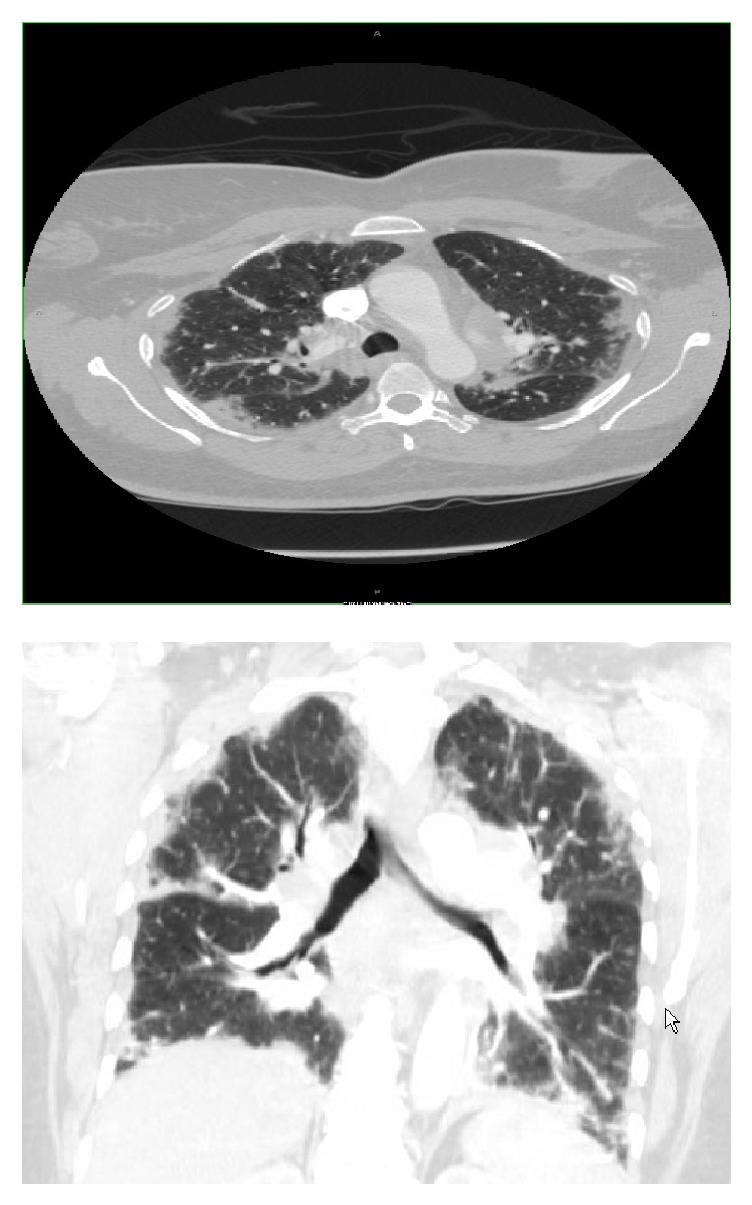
Chest computed tomography (CT) scan showing increased bilateral interstitial lung markings with bilateral hilar and mediastinal lymphadenopathy most consistent with stage IV sarcoidosis.
